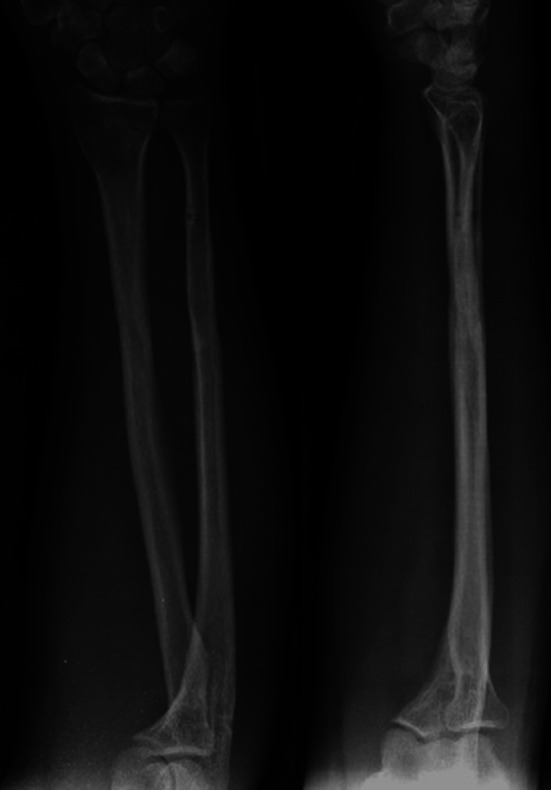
AP and lateral radiographs showing implant removal 20 months after surgery
